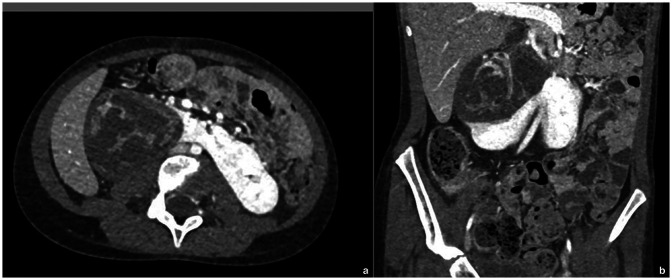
Volumetric decrease of the size of the tumor in an enhanced CT scan. (a) Axial contrast enhanced abdomen CT scan (venous phase): volumetric decrease of the solid tumor arising from horseshoe kidney’ side (6.8 × 5.2 × 6.6 cm vs. 13.5 × 8 × 11 cm) after neo-adjuvant chemotherapy. Hypodense intralesional areas show necrotic effect after treatment. (b) Coronal contrast-enhanced abdomen CT scan (venous phase): volumetric decrease of the mass shows a decreased mass effect on the IVC and the abdominal aorta but residual compression on the remnant kidney parenchyma.
